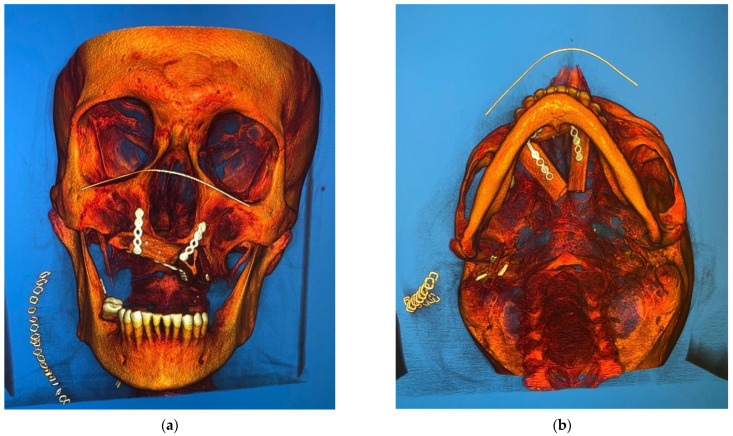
CBCT reconstruction after surgery (a-frontal view; b-coronal view). The CBCT was performed the day after surgery.
